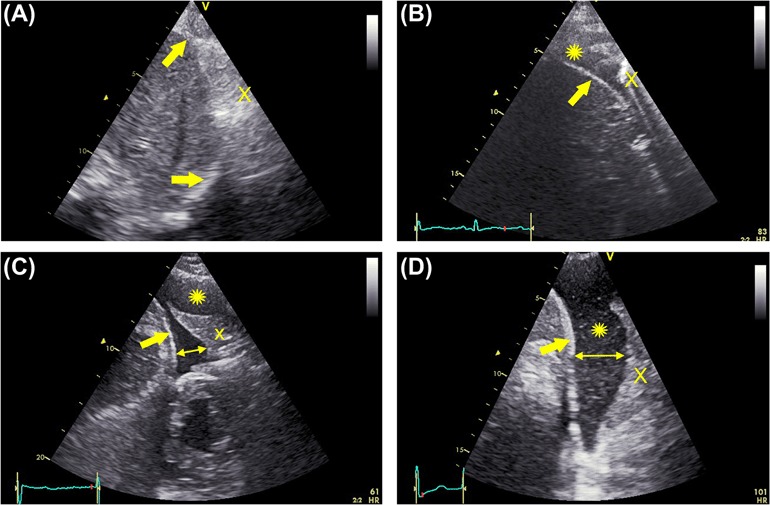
Chest ultrasound with examples of pleural effusion. A: absent, B: small (only in the costophrenic angle), C: moderate, D: large. *Double arrows* show the measurement of pleural effusion. *Thick arrows* indicate the diaphragm. X: lung (in B air-filled, in C and D consolidated). ∗pleural effusion.
